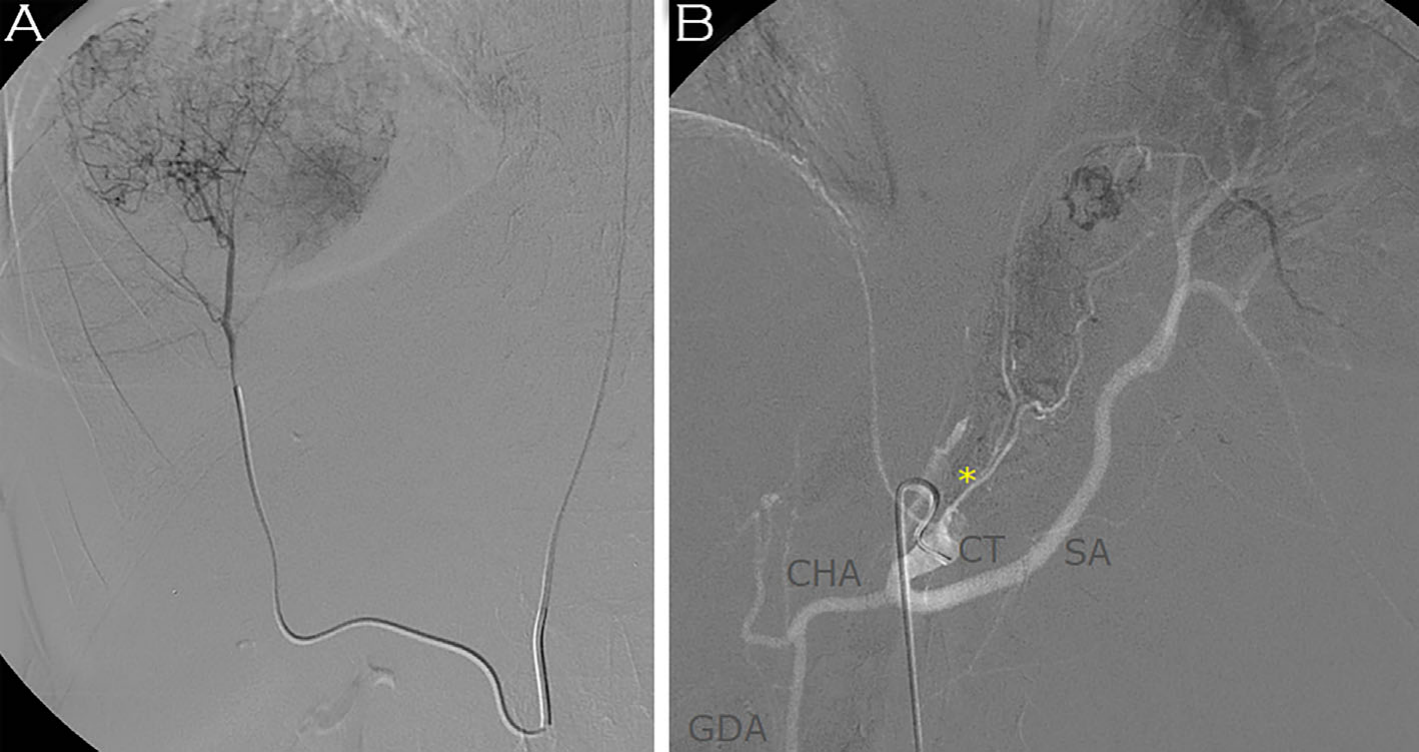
Embolization of the S8 tumor through the right replaced hepatic artery was achieved with 0.1mm PVA particles (A). Embolization of the primary tumor through the left inferior phrenic artery was performed by 0.2mm PVA particles (B). CT, celiac trunk; SA, splenic artery; CHA, common hepatic artery; GDA, gastroduodenal artery; *, dominant suprarenal artery from left inferior phrenic artery.
